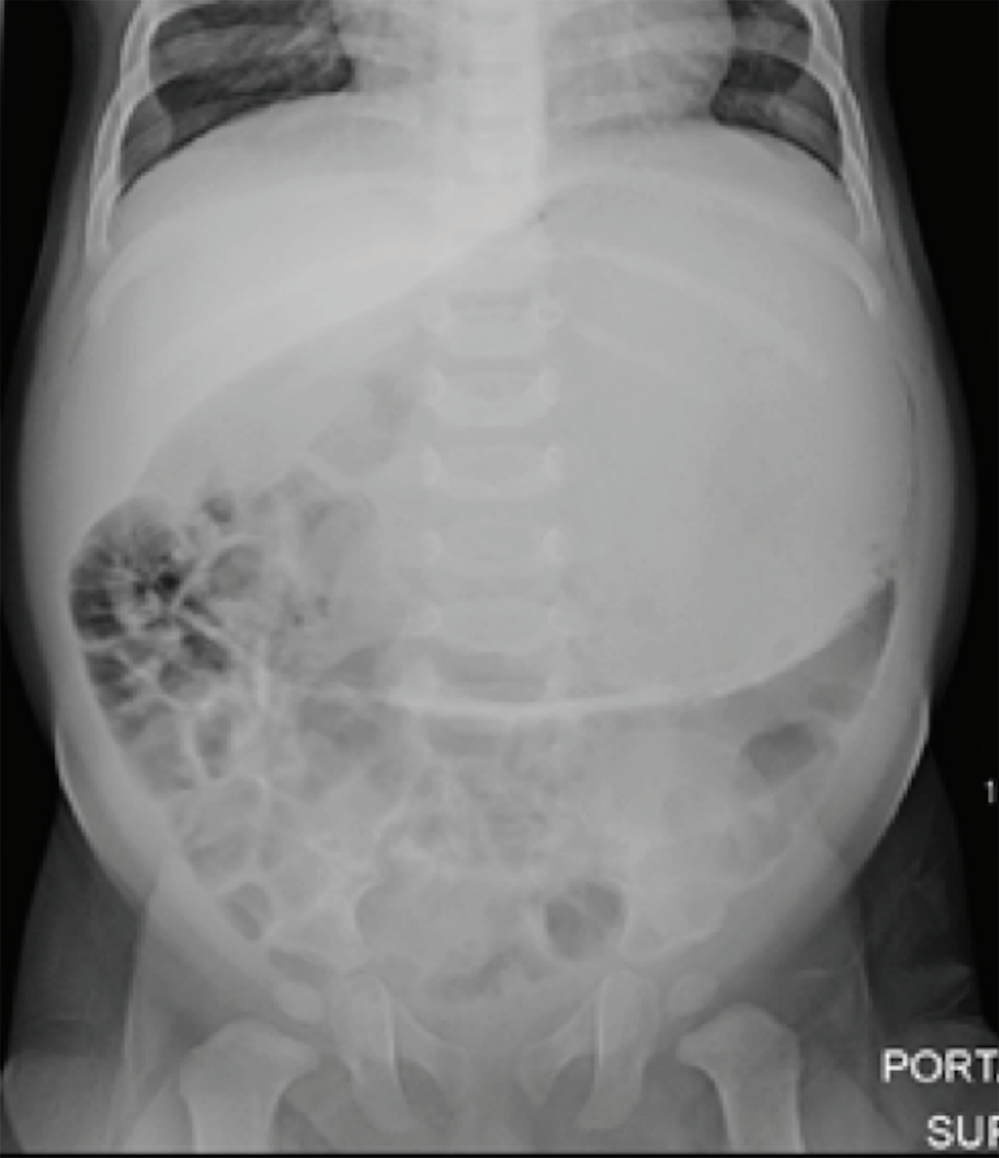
Kidney, ureter, and bladder (KUB) radiograph revealing severe gastric distension with normal bowel gas pattern concerning for gastric volvulus
